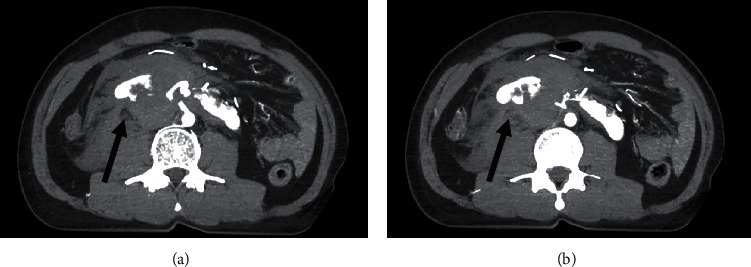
CT angiography in axial view, showing an active contrast extravasation from accessory right renal artery and retroperitoneal hematoma (a, b) (black arrow).
